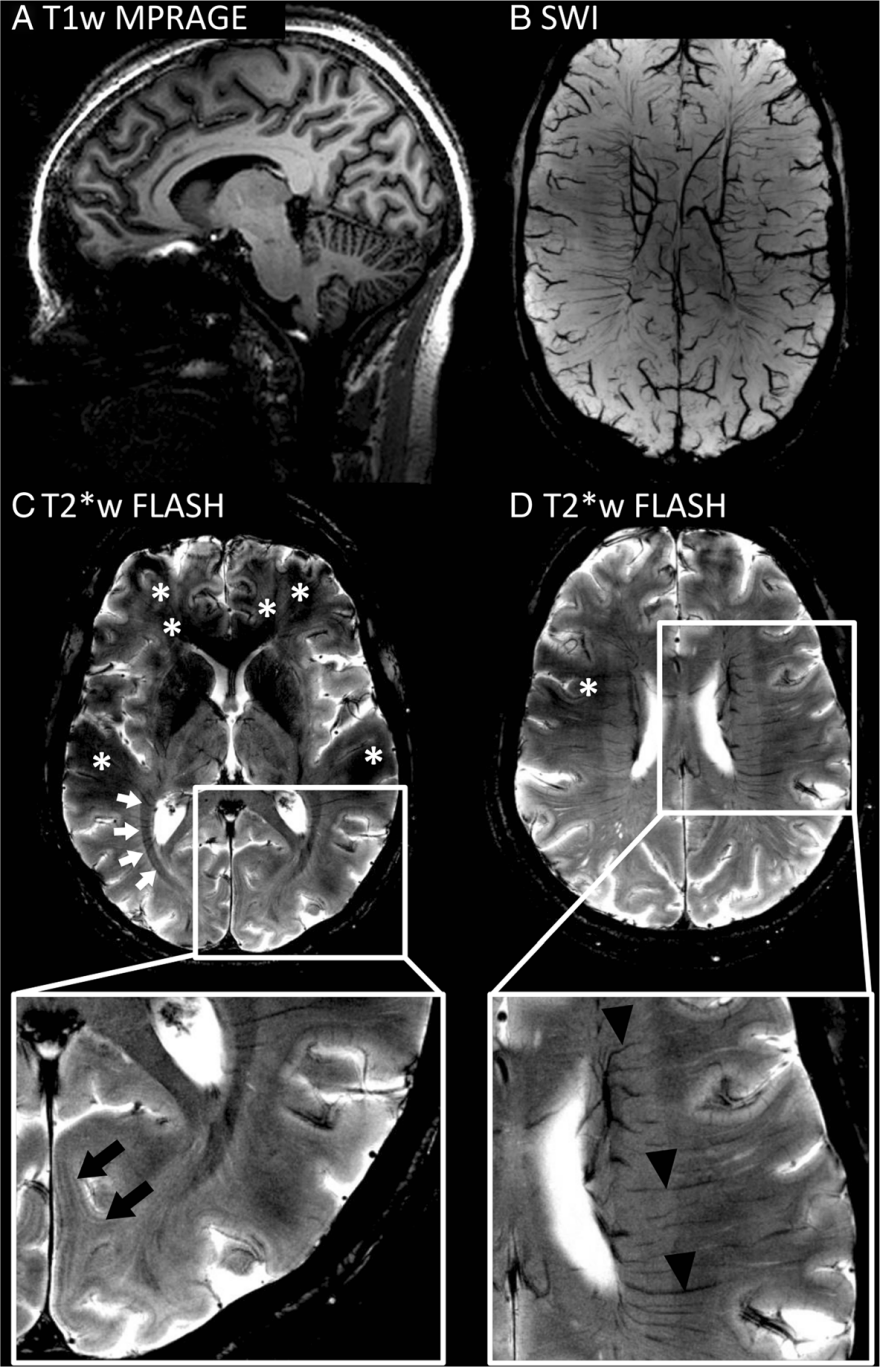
Brain structures visualised on 7 Tesla MRI images. a 7 T T1w MPRAGE provides high-resolution anatomical imaging with excellent gray to white matter contrast. b 7 T SWI depicts very small brain veins. c, d 7 T T2*w FLASH with a resolution of 0.2 mm × 0.2 mm × 2 mm delineates strongly myelinated structures such as the optic radiation (*white arrows*) or the stripe of Gennari (*black arrows*, zoom). In addition, very small veins are visualised in the periventricular white matter (*black arrowheads*, zoom). Nevertheless, the image quality of 7 T gradient echo images is sometimes reduced due to inhomogeneities or artifacts (*asterisks*)
